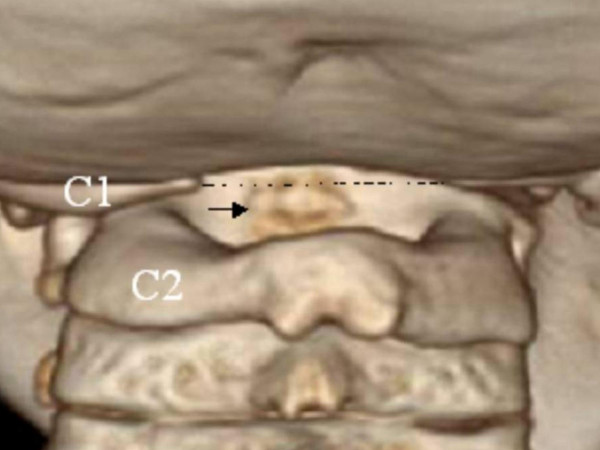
3D reconstruction CT scan showing oval shaped os odontoideum in relationship to the anterior normal arch of the atlas (arrow a); there is marked hypoplasia of the posterior arch of the atlas (arrow b).
